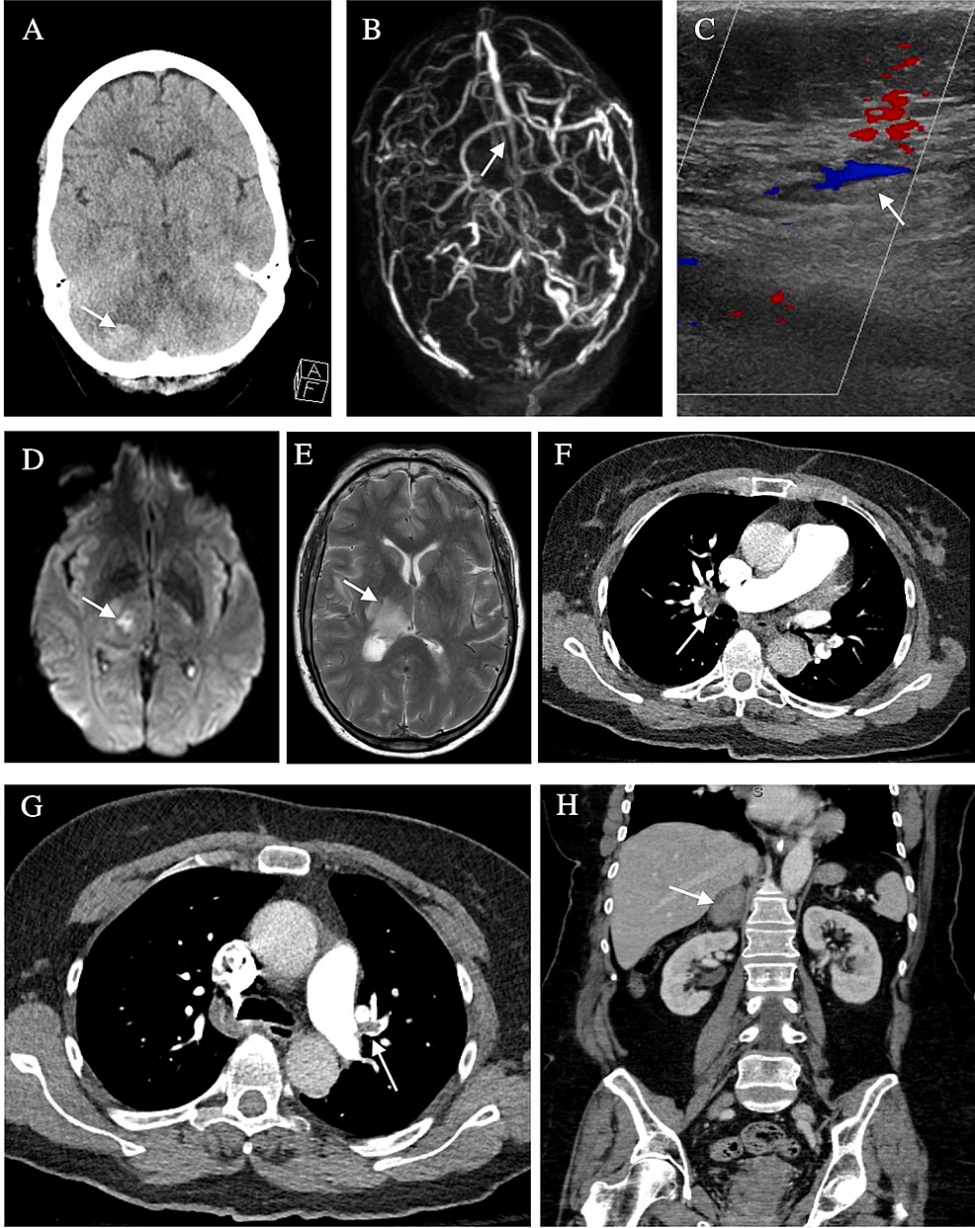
Radiographic Images of Multiorgan Thrombosis and Hemorrhage Select radiographic imaging of the patient's workup during the third hospitalization. Arrows point to pathology where appropriate. A) Axial CT brain showing right cerebellar hemorrhage. B) 3D reconstruction of the MRV brain showing superior sagittal thrombosis and right transverse sinus thrombosis. C) Lower extremity venous doppler of the left posterior tibial vein showing age-indeterminate venous thrombosis. D) MRI brain DWI sequence showing diffusion restriction of the right cerebral peduncle. E) MRI brain T2 sequence showing right thalamic hemorrhage. F) Axial CTA chest showing right segmental pulmonary embolism. G) Axial CTA chest showing left pulmonary artery embolism. H) Coronal CT abdomen and pelvis with contrast showing large subacute right adrenal hematoma.
